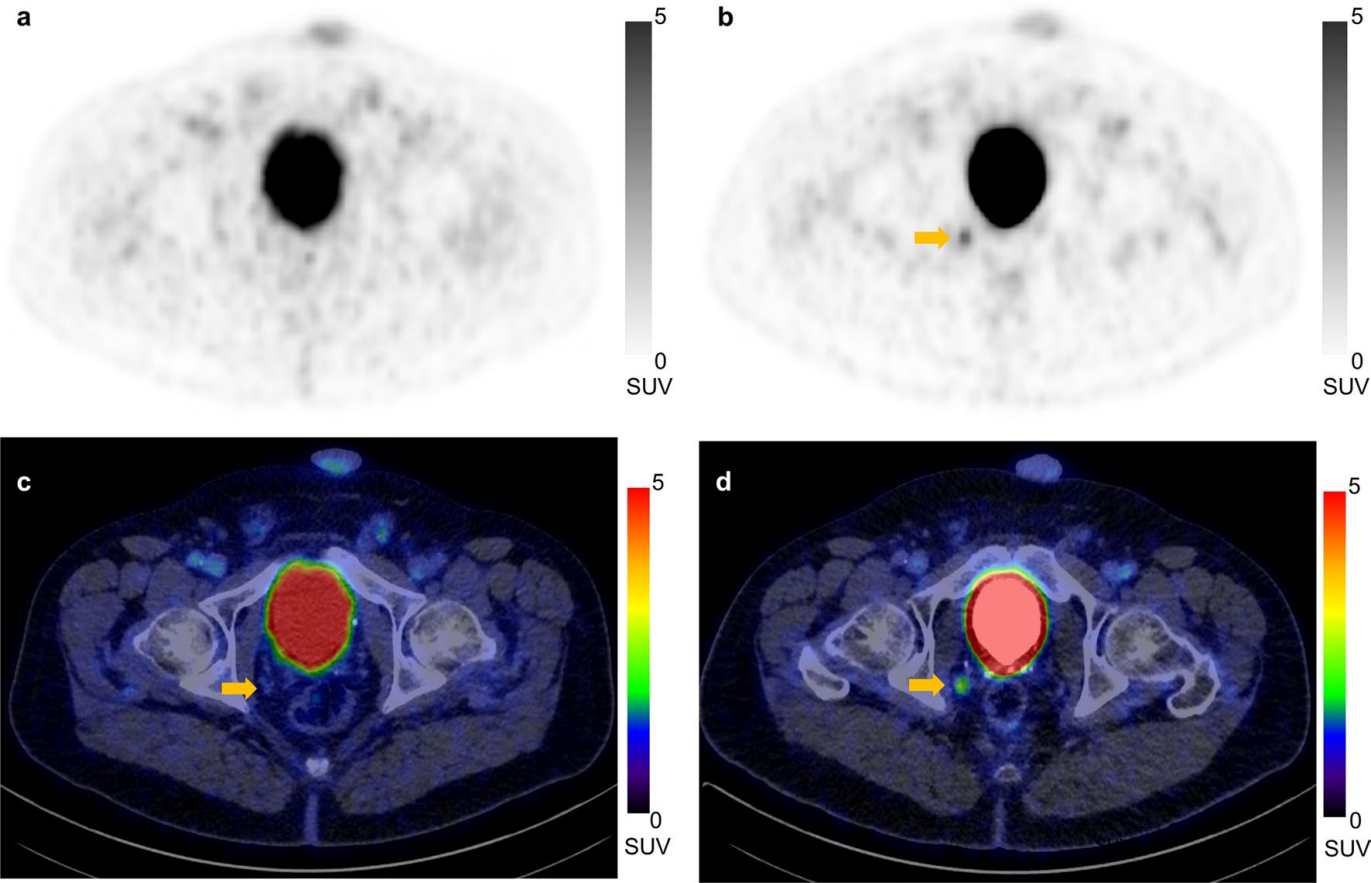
PET (a, b) and PET/CT fusion c, d images of the pelvis with increasing uptake of [.^68^ Ga]Ga-PSMA-I&T of a lymph node (arrows) in the deep internal iliac lymph node region on the right side (early scans: a and c; late scans: b and d)
